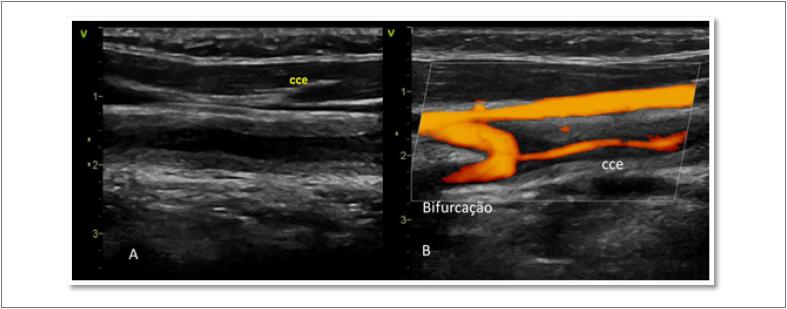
Arterite de Takayassu, sexo feminino, 17 anos de idade. A. Corte longitudinal da artéria carótida comum esquerda mostra importante espessamento concêntrico da parede. B.119 Imagem ao powerDoppler com grande redução luminal da artéria carótida comum, com lúmen preservado a partir da bifurcação carotídea.
